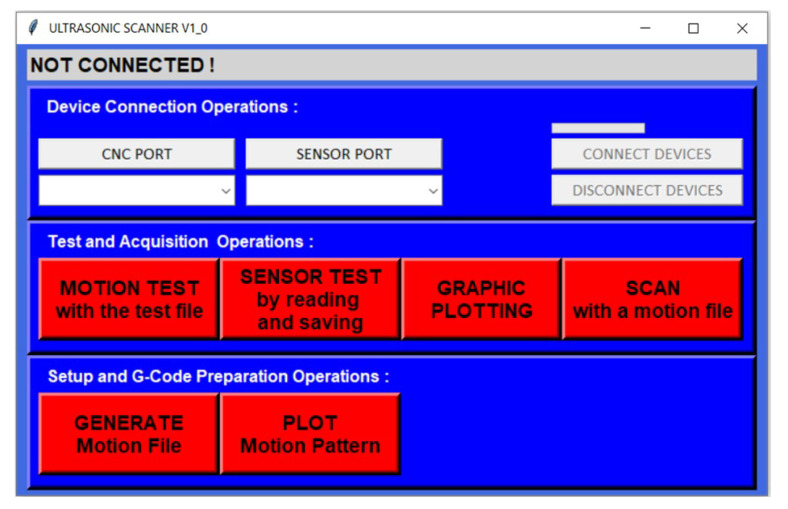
Improved ultrasonic scanner user interface.
